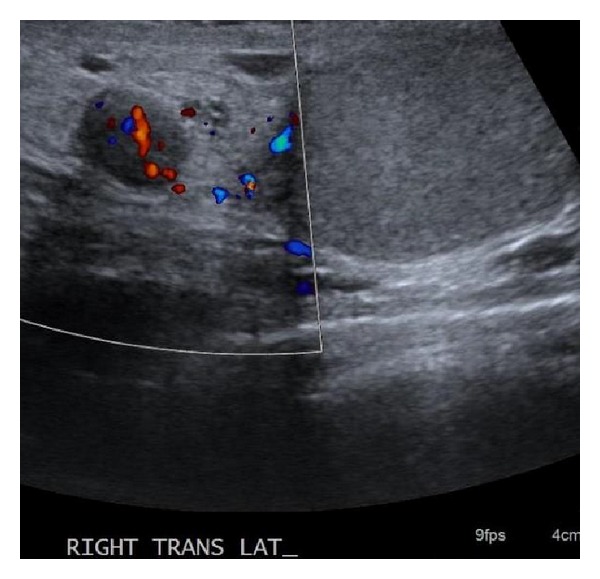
Scrotal sonogram demonstrating a 0.7 × 0.8 × 0.6 cm hypoechoic, vascular, heterogeneous lesion superior to the normal right testicle.
